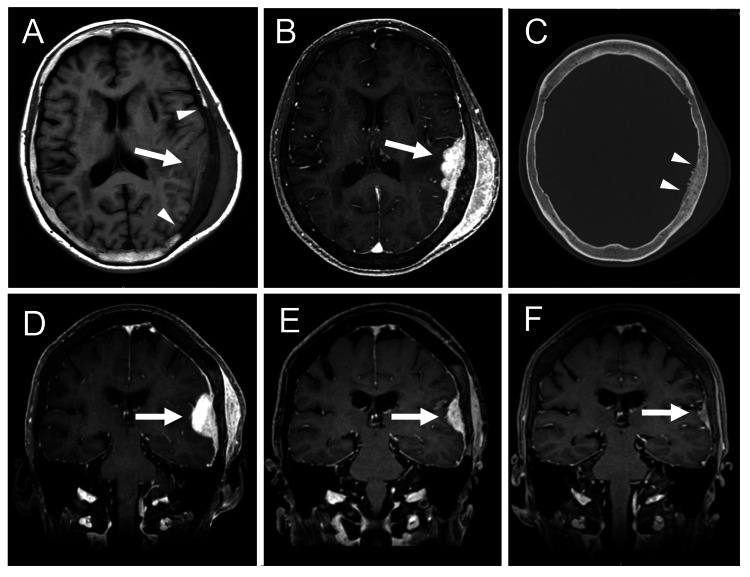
MR imaging and CT scan in the clinical course. (A) An axial slice of T1-weighted MR image at the initial visit. An arrow indicates an isointense mass lesion attached to the cranial vault. Arrowheads indicate clear boundaries of the lesion in the bone marrow. (B) An axial slice of gadolinium-enhanced MR image at the initial visit. An arrow indicates the lesion extending intracranially and extracranially and well-enhanced with gadolinium. (C) A CT scan of the cranial bone at the initial visit. Arrowheads indicate hyperostosis in the inner surface of the bone involved in the lesion. (D-F) Coronal sections of MR images obtained at the initial visit (D), a few weeks later (E), and at the latest follow-up (F). Arrows indicate the lesion in the cranial vault that decreases in size spontaneously.
